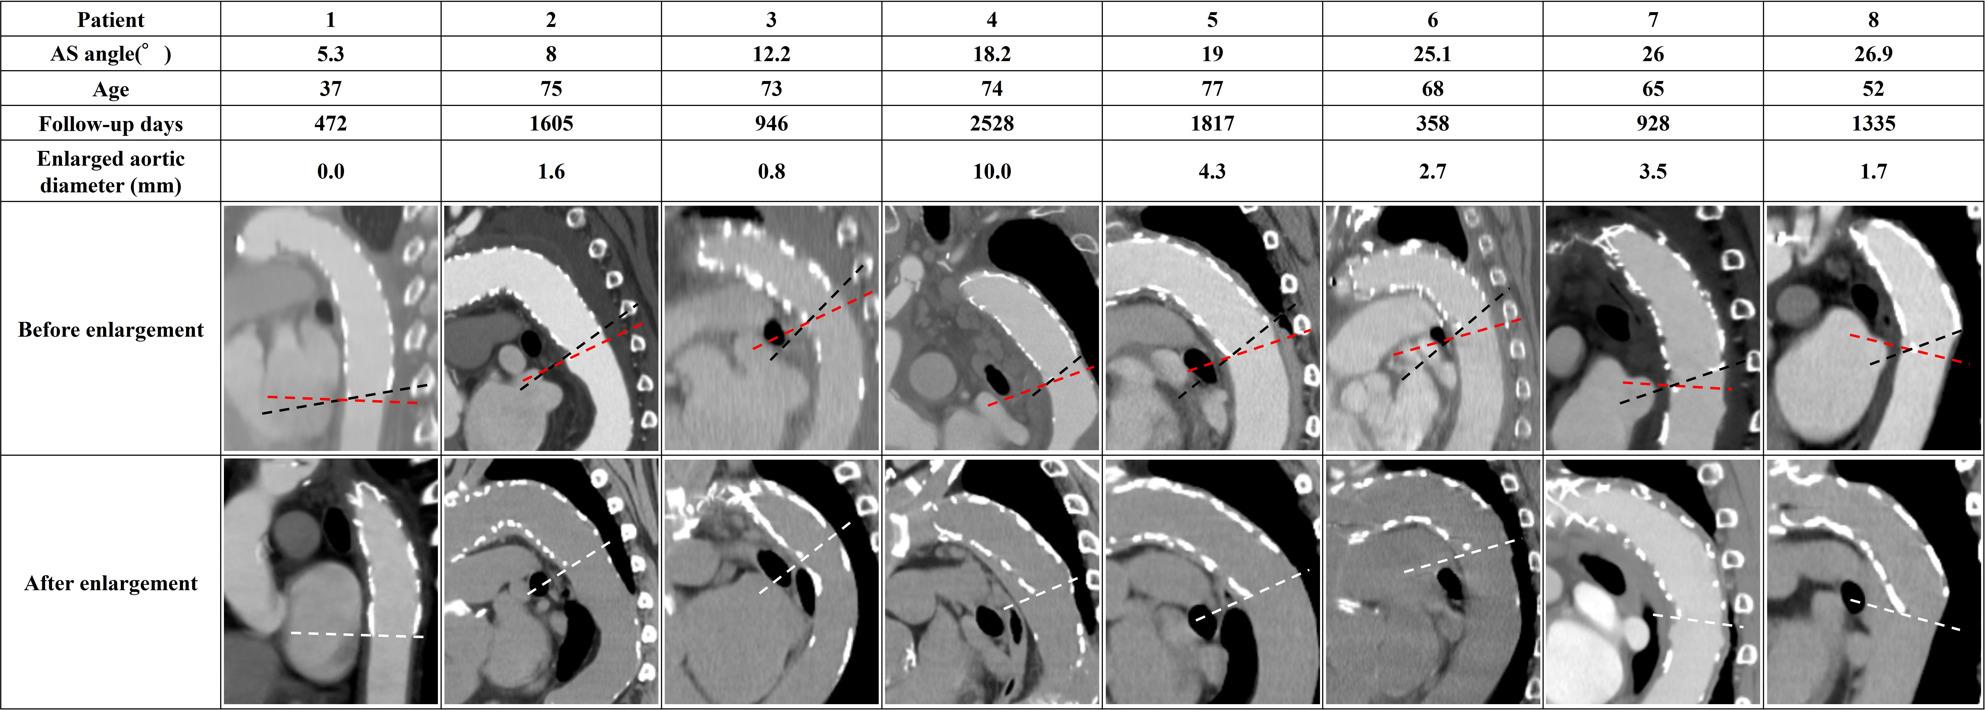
Patient-specific AS angles and CT views (Patients 1–8). AS angle is formed by the red and black dashed lines. Enlarged aortic diameter was defined as the difference between the post-enlargement plane (white dashed line) and the pre-enlargement plane (red dashed line) at the distal FET level. AS angle, aorto-stent graft angle
